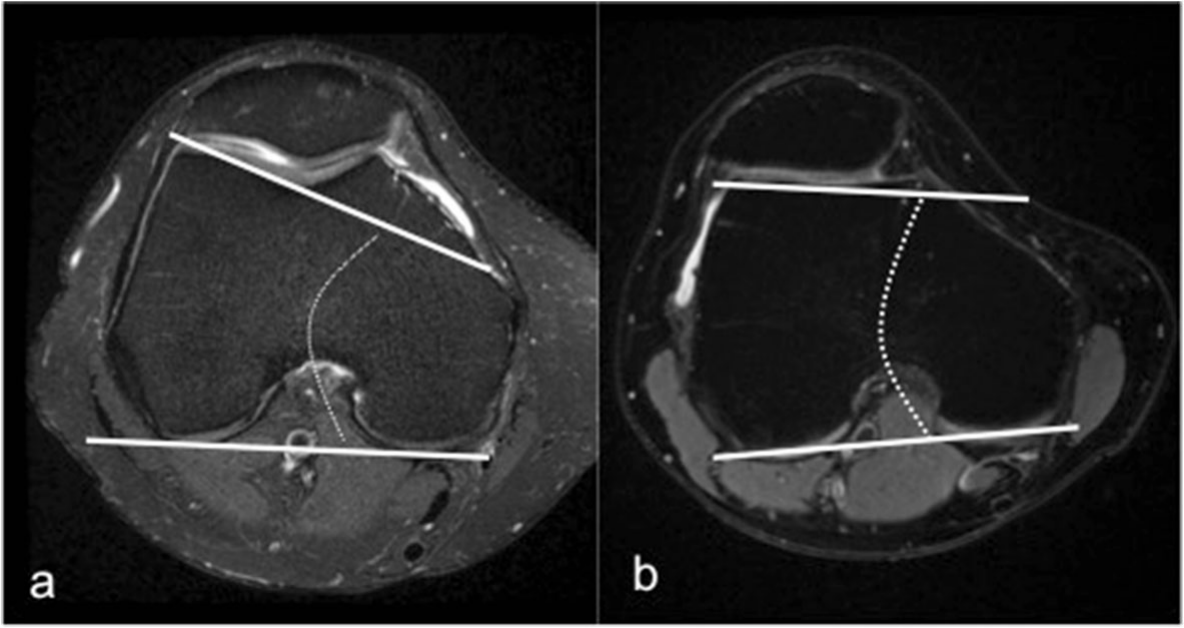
Lateral trochlear inclination measurement on axial MRI. It is the angle between a line tangential to the subchondral bone of the posterior aspect of the femoral condyles and a line along the lateral trochlear facet. a Normal trochlea. b Trochlear dysplasia (9° inclination)
